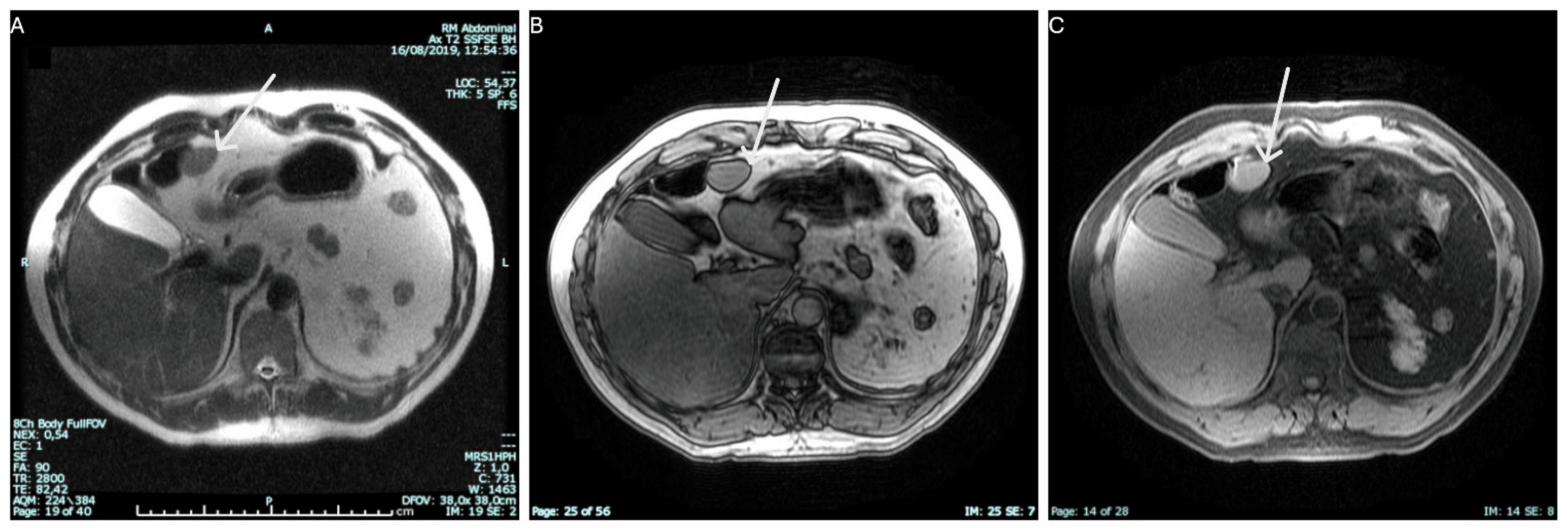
Axial T2 image without fat suppression (A), T1-weighted outphased image, and (B) T1-weighted FatSatFSPGR image(C) The lesion is homogeneous and isointense relative to the liver parenchyma on T2 and T1 weighted images and has no signal drop on T1 outphased images. FatSatFSPGR: fat-saturated fast spoiled gradient-echo
